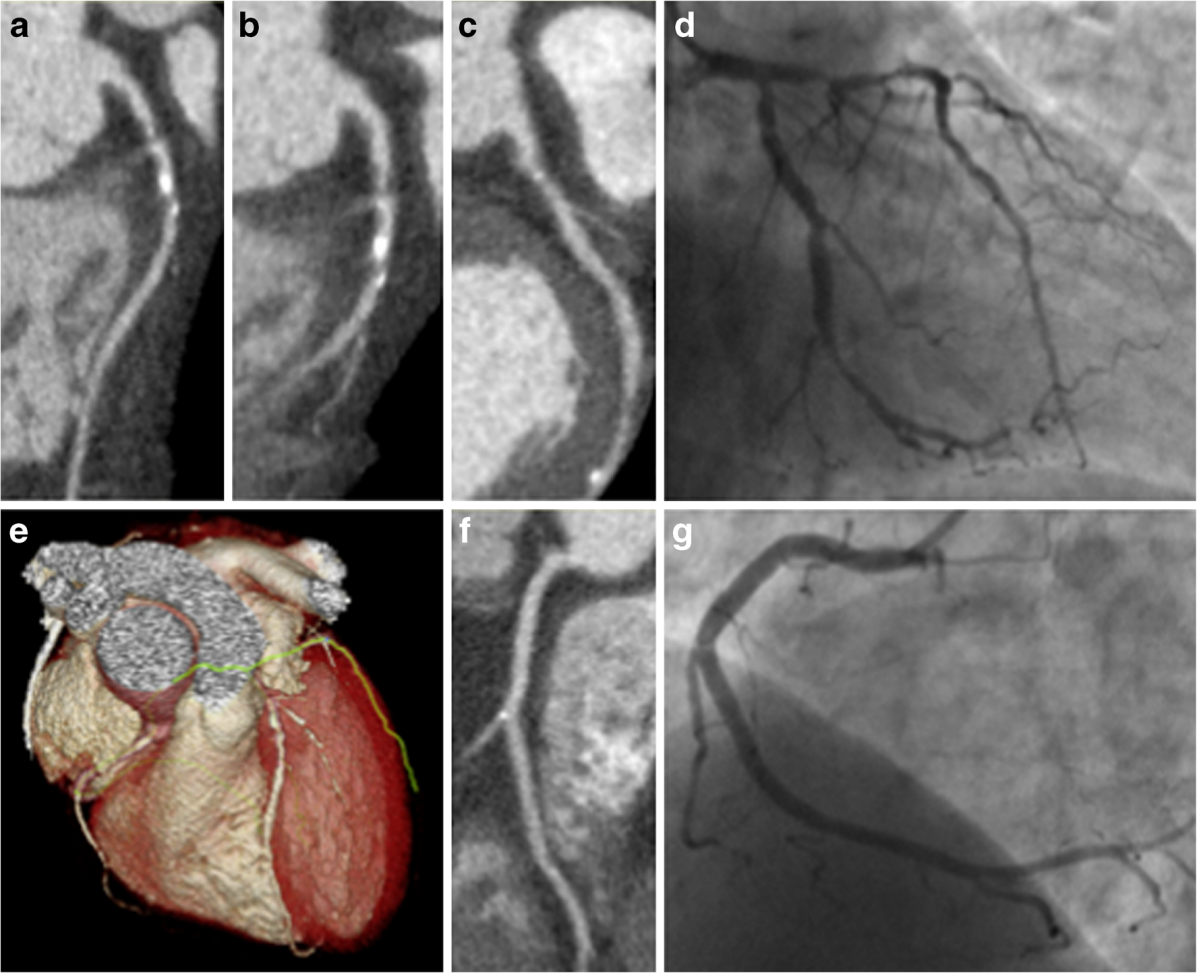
An example of low radiation dose CCTA with comparison to invasive coronary angiography from the SCOT-HEART study. This 60-year-old male patient with no previous history of cardiovascular disease and no cardiovascular risk factors presented with chest pain. CCTA identified severe disease in the left anterior descending coronary artery (a), first diagonal (b) and circumflex artery (c) which was confirmed on invasive coronary angiograph (d). Mild non-obstructive disease was identified in the right coronary artery (f, g). e Shows a three-dimensional reconstruction of the heart from the CT. The patient underwent revascularisation. The total dose length product for CCTA and calcium scoring CT was 358 mGy cm (5 mSv using the 0.014 mSv/mGy cm conversion factor)
